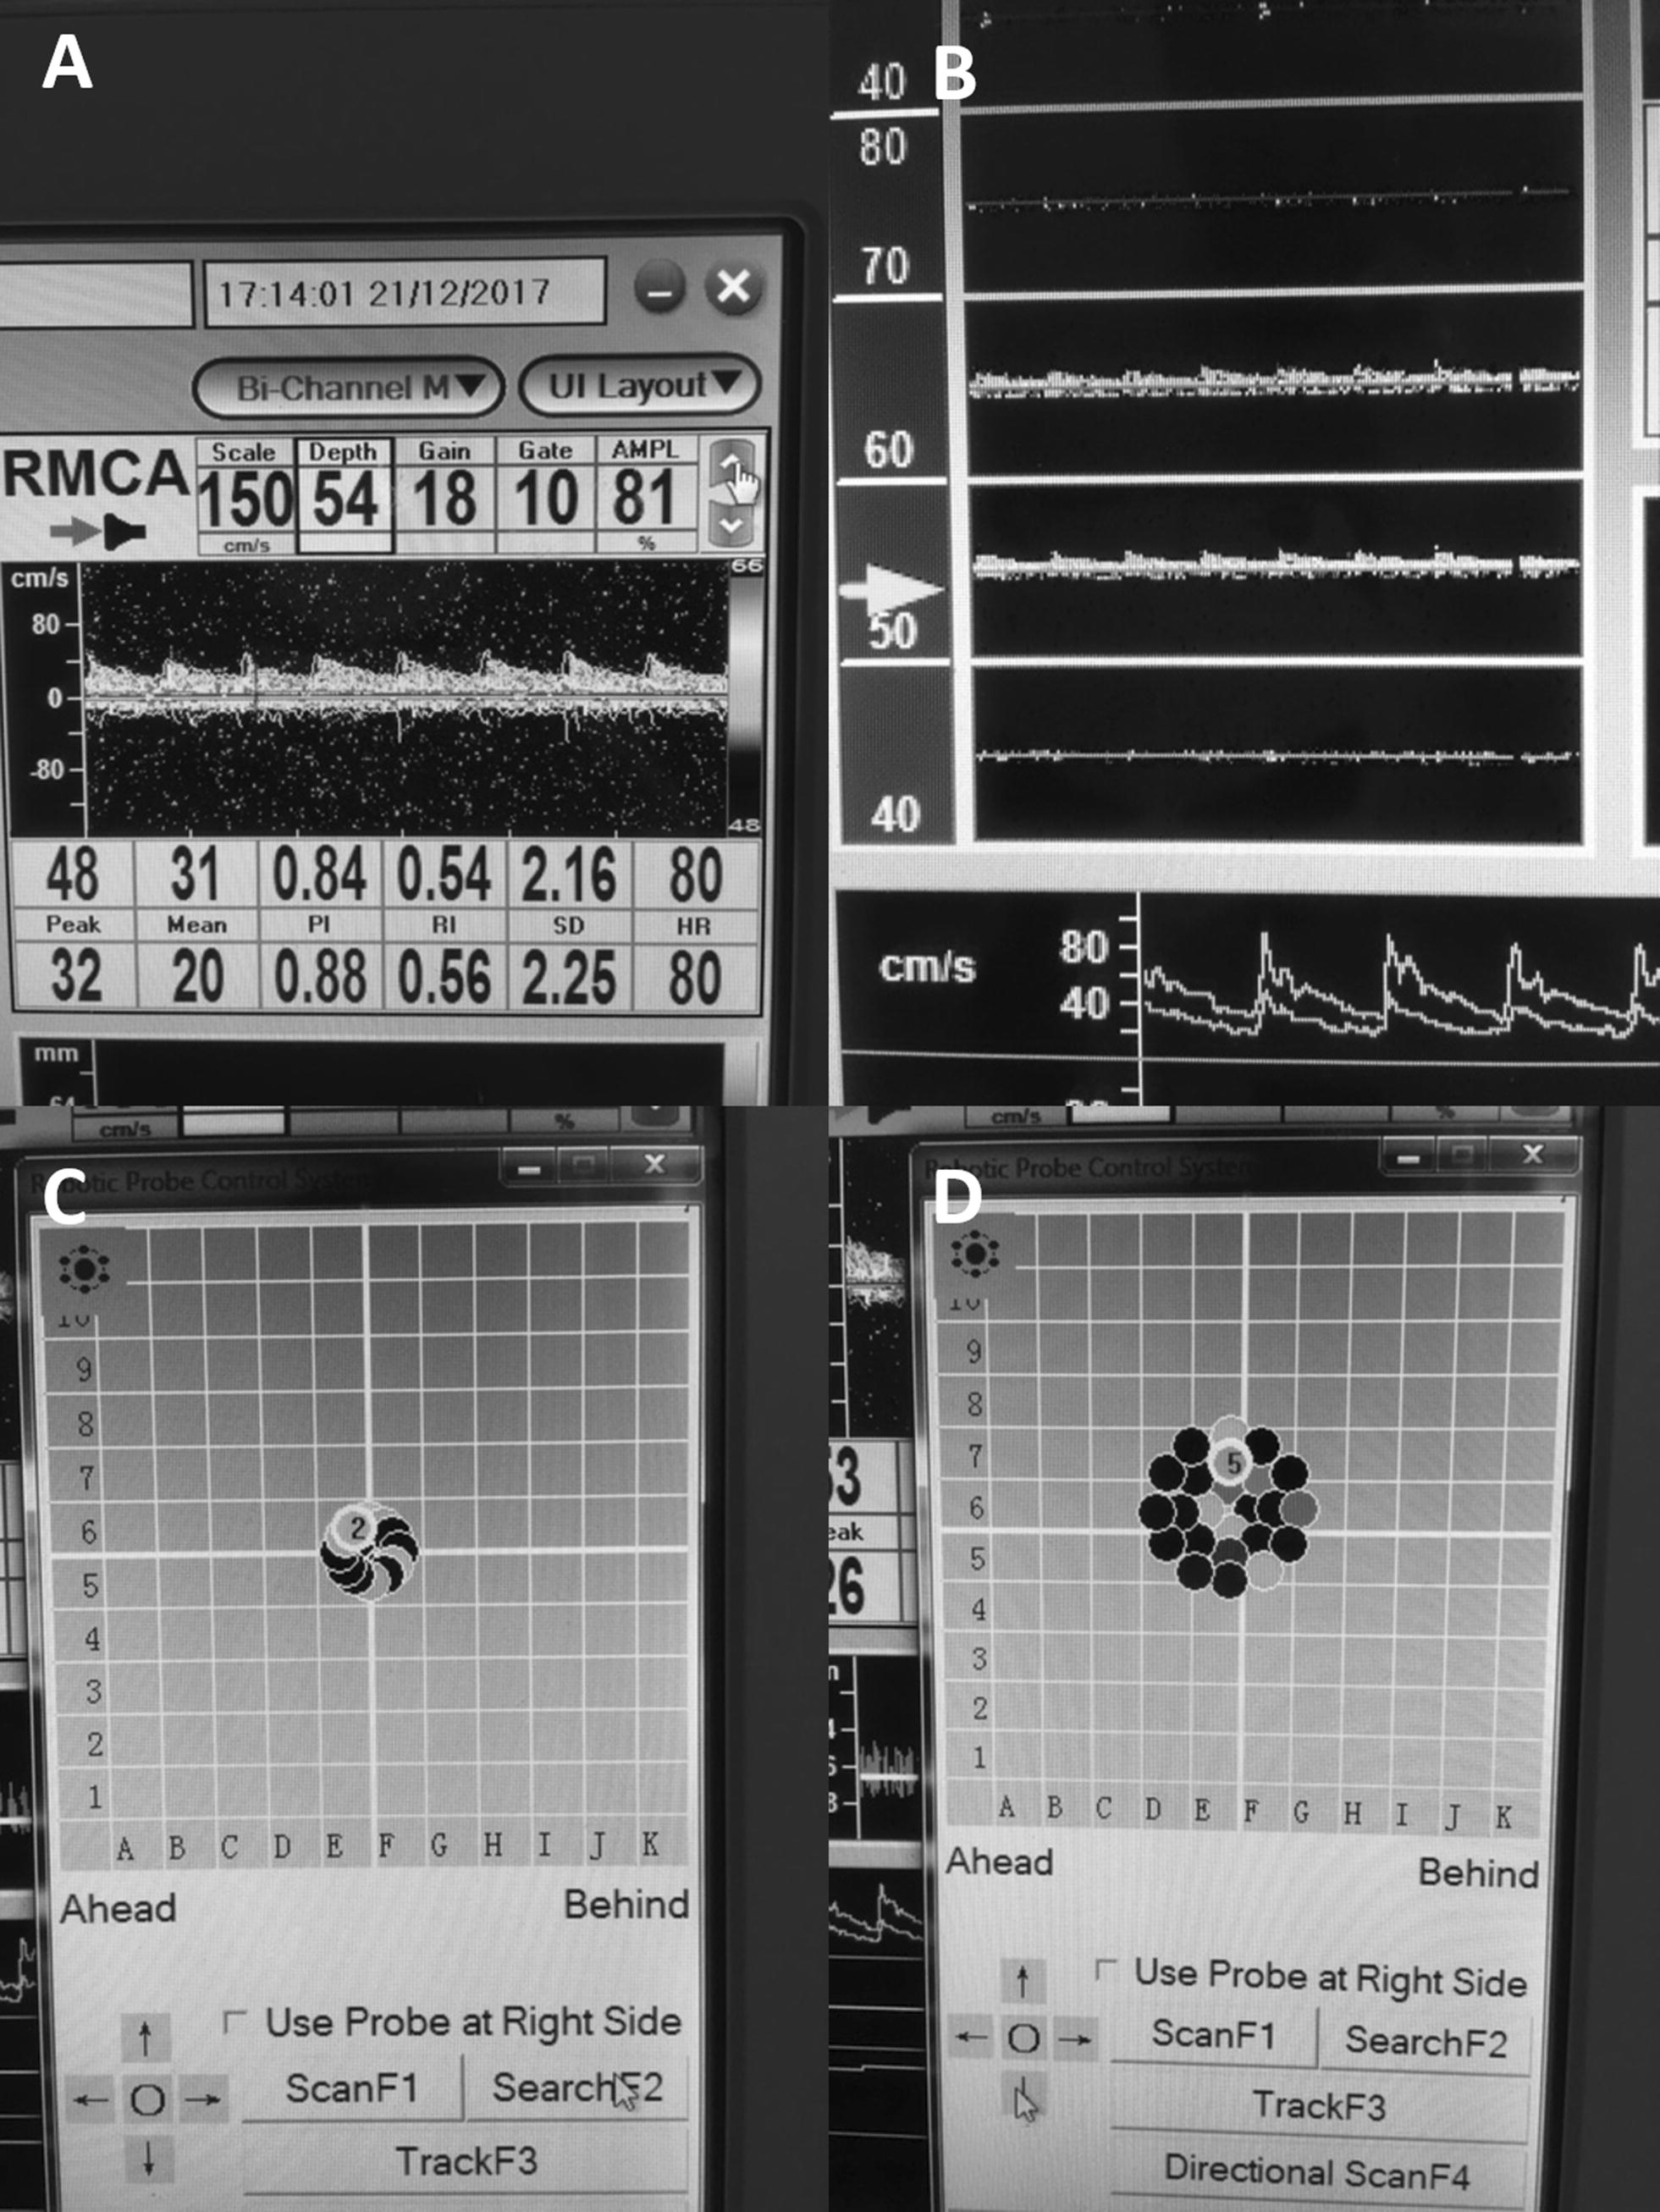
Delica EMS 9D TCD software interface. *TCD* transcranial Doppler. A Displays right middle cerebra artery real-time blood flow velocity waveform. B Displays sequential “look ahead” view of right middle cerebral artery, providing sequential real-time cerebral blood flow velocity waveforms at various depths of insonation (in this panel the presence/absence of flow velocity waveforms can be seen at depths of 45, 55, 65 and 75 mm in front of the probe face). C Search robotic signal acquisition function window, displaying the pattern at which the TCD probe is automatically moved. D Directional robotic signal acquisition function window, displaying the pattern which the TCD probe is moved, changing the angle of insonation. C and D, the various points of insonation are color coded to represent strength of signal, this can be appreciated via different shadings in this greyscale picture
